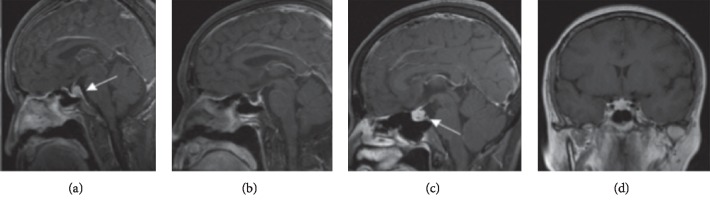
Dynamic magnetic resonance imaging (MRI) of the pituitary (T1-weighted). Enlargement of the posterior pituitary lobe and absence of the hyperintense signal of the posterior pituitary gland on T1-weighted images of patient No. 1 (a). Reappearance of posterior pituitary bright spot and the mass of the posterior pituitary on T1-weighted images of the same patient almost completely resolved after treatment (b). Enlargement of a space-occupying lesion and loss of the normal posterior pituitary T1 hyperintense signal on dynamic MRI of the pituitary (T1-weighted) of patient No. 2 (c, d).
